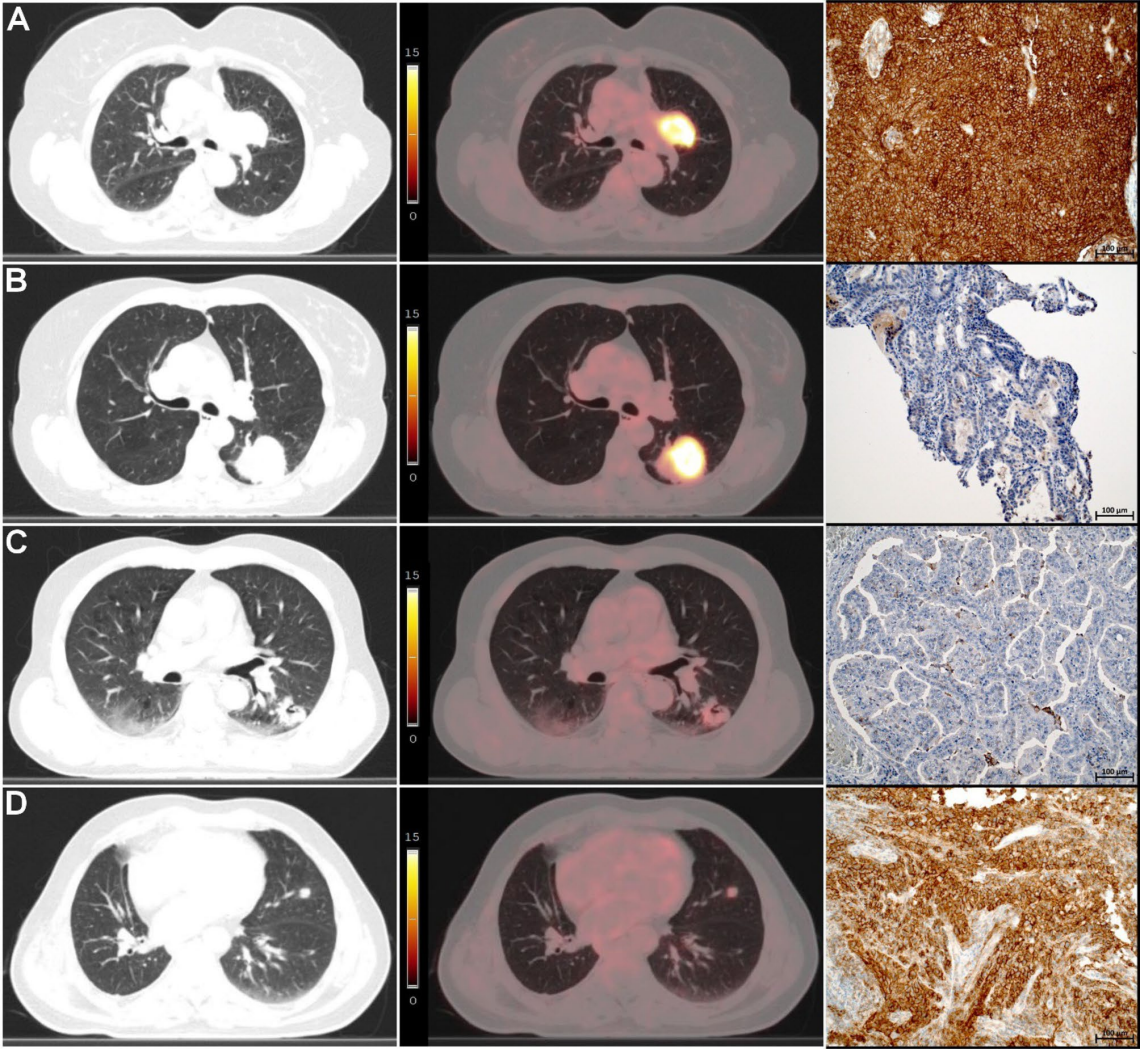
Examples of primary lung cancers demonstrating various levels of metabolic activity (SUVmax) vs. PD-L1 expression (TPS). Row A - SUV_max_ =26.5, TPS = 95%; row B - SUV_max_ =21.6, TPS < 1%; row C - SUV_max_ =4.4, TPS < 1%; row D - SUV_max_ =3.1, TPS = 85%
